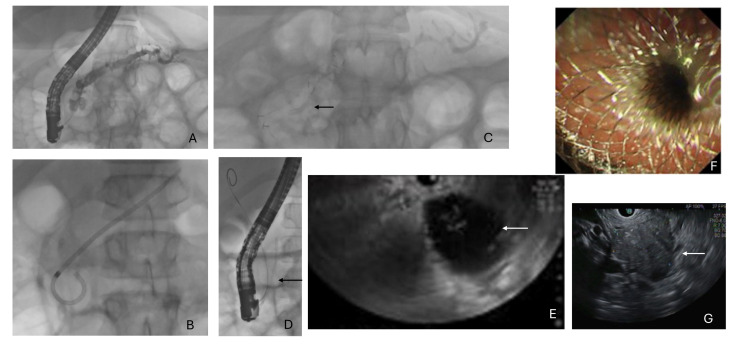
Endoscopic retrograde cholangio-pancreatography (A–D) and endoscopic ultrasound (E–G). (A) Main pancreatic stricture with a pancreatic duct stone, (B) plastic stent, (C) fully covered metallic ‘Bumpy’ stent (black arrow), (D) Archimedes biodegradable stent (black arrow), (E) pancreatic fluid collection (white arrow), (F) endoscopic view following successful deployment of 8 × 8 mm LAMS to drain a symptomatic pancreatic fluid collection, (G) head of pancreas mass (white arrow) in a patient that underwent a fine needle biopsy and received a subsequent diagnosis of autoimmune pancreatitis.
